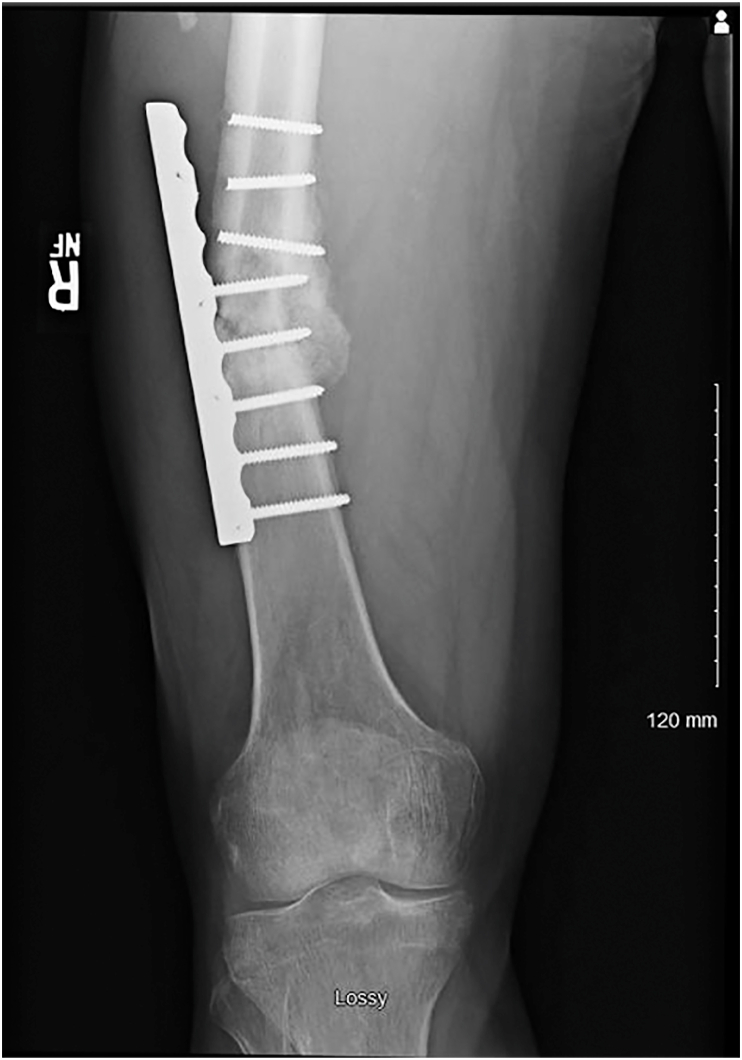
Right femur anteroposterior radiograph depicting catastrophic hardware failure of the lateral plate and screws with subsequent oligotrophic nonunion.
